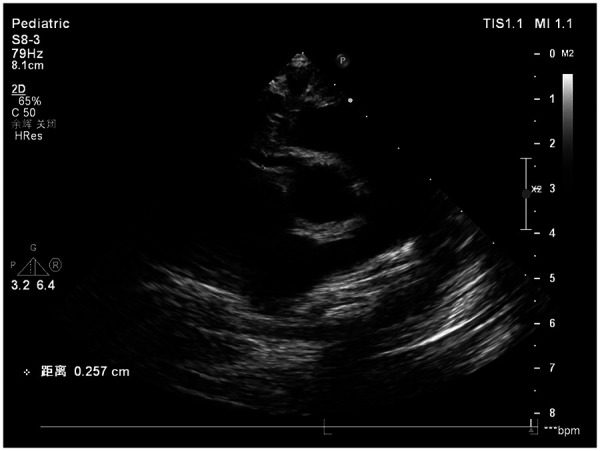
Echocardiography on the 4th day of hospitalization (right coronary artery *Z*-score 2.06). This echocardiogram shows mild dilation of the right coronary artery (*Z*-score 2.06) and no obvious abnormalities of the left coronary artery in the child on the 4th day of hospitalization (5th day of illness), suggesting early coronary artery involvement. *Z*-scores were calculated using the Canadian reference equations (16).
